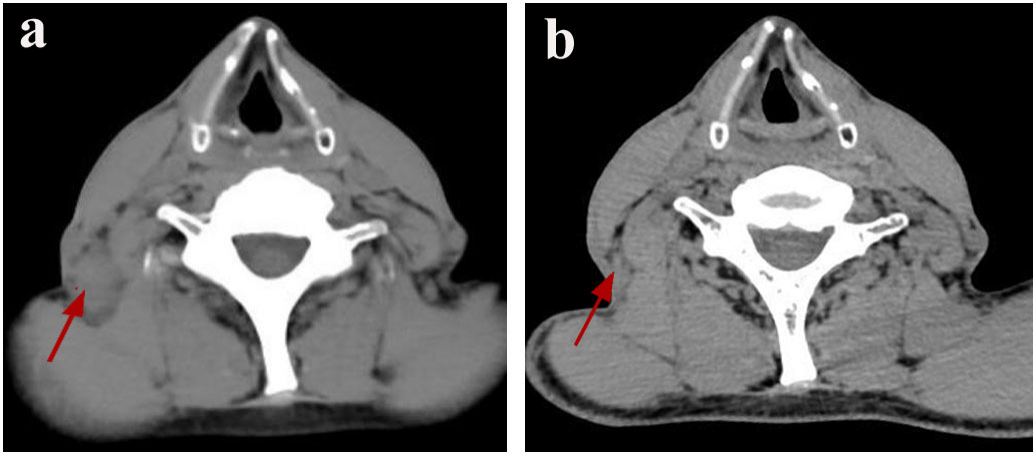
Resolution of CSD-associated lymphadenopathy on serial cervical CT. (a) Pre-treatment CT scan (January 8, 2025) demonstrates an enlarged right posterior cervical lymph node (red arrow). (b) Follow-up CT scan (March 26, 2025) shows the enlarged lymph node in the right posterior cervical space has disappeared (red arrow).
